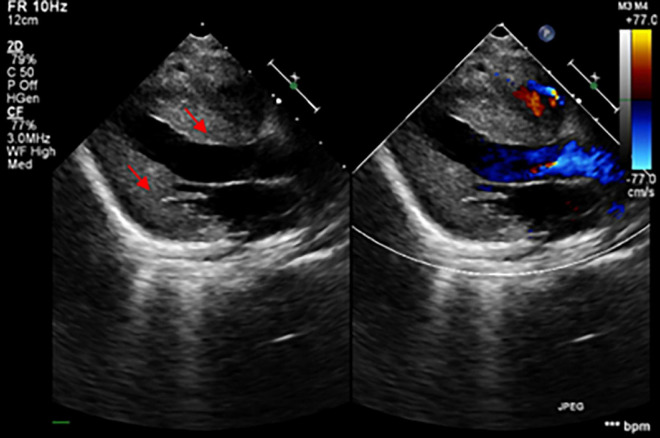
The echocardiogram demonstrated the thickening of the myocardium (the red arrows showed the hypertrophic interventricular septum and posterior wall of the left ventricle).
